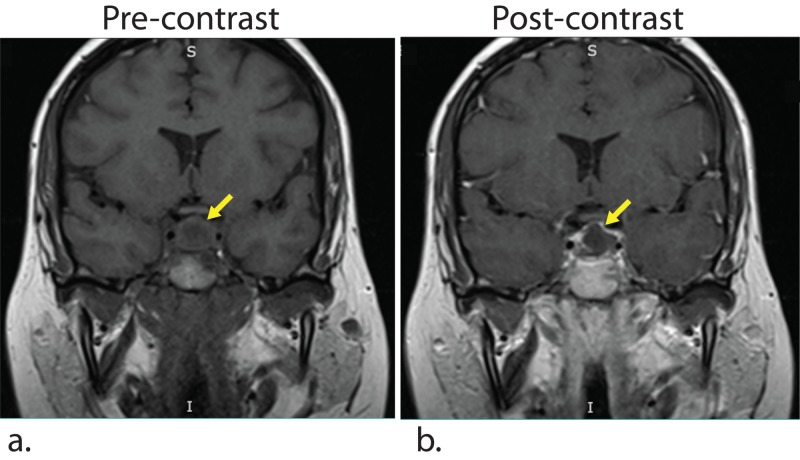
MRI 10 months prior to admission (a) Pre-contrast T1-weighted MRI image demonstrates a 1.2 x 1.6 x 1.5 cm (AP x TR x CC) lesion in the sella turcica, which is thought to be Rathke’s cleft cyst (yellow arrow). (b) Post-contrast T1-weighted MRI image shows lack contrast enhancement. AP, anteroposterior; TR, transverse; CC, craniocaudal.
